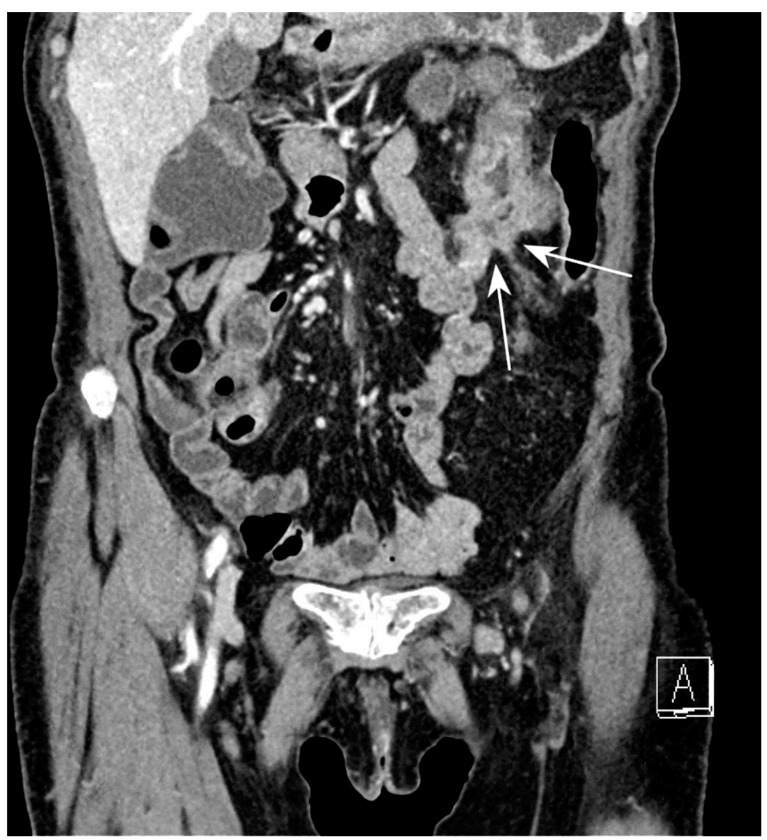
Entero-enteric fistula: CT-E coronal image after iodinated contrast injection and administration of polyethylene glycol solution shows entero-enteric fistula between the descending colon and an adjacent ileal loop (arrows). Letter “A” indicates the coronal view of the CT-E image. Original figures by LMM and LL.
